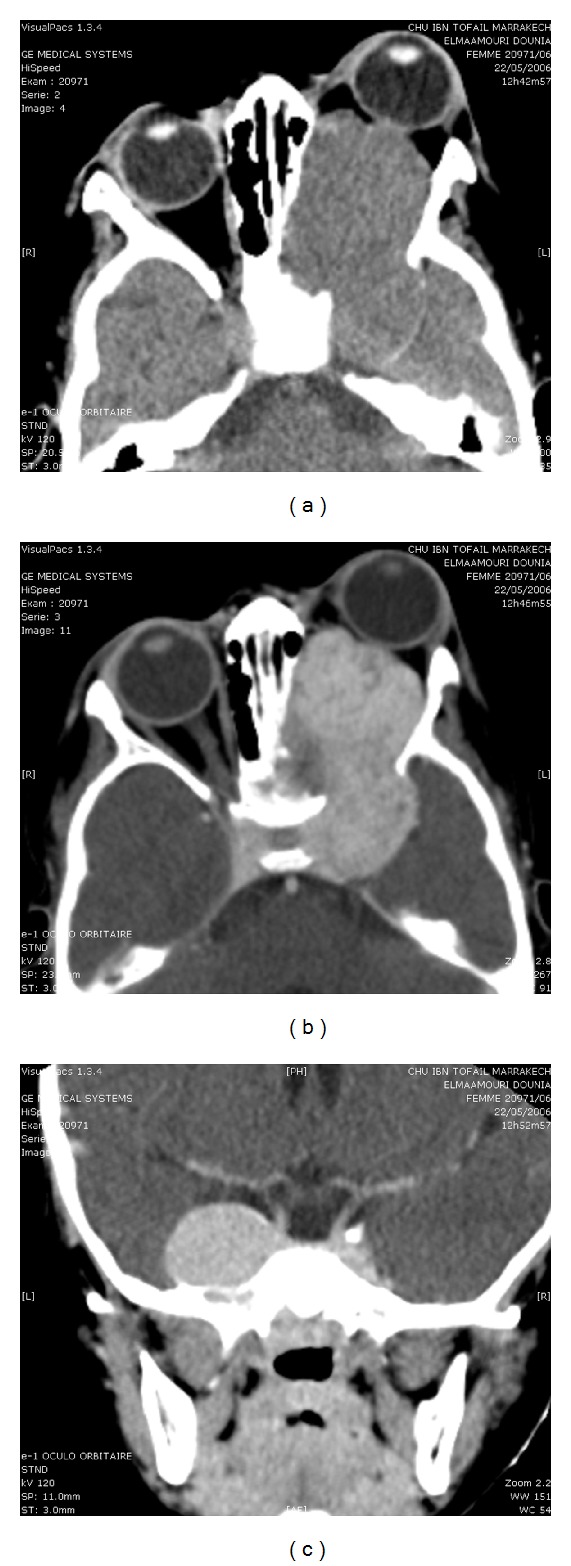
CT scan on axial (a) and coronal (b) views with contrast administration shows an endoorbital tumor involving the optical nerve spreading to the cavernous sinus and widening the pterygopalatine fissure.
